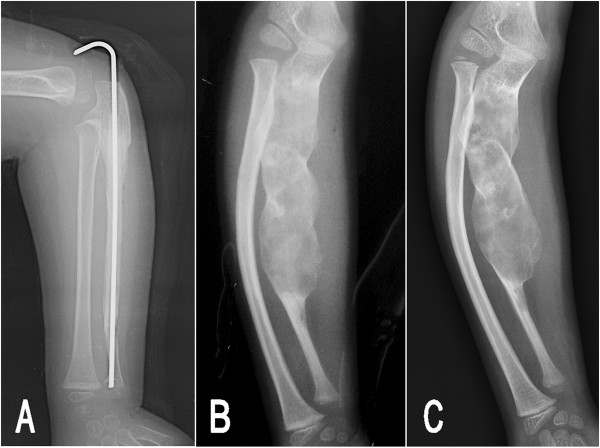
Postoperative radiographs of patient 1. At 6 weeks postoperation, the lateral radiograph of the right forearm (A) showed a well grafted union. At 4 years of follow-up (B), the lesion had recurred to an enlarged extent with bowing deformity in both the ulna and radius, and the lesion and deformity continued to be progressive over time (C) at 8 years postoperation.
